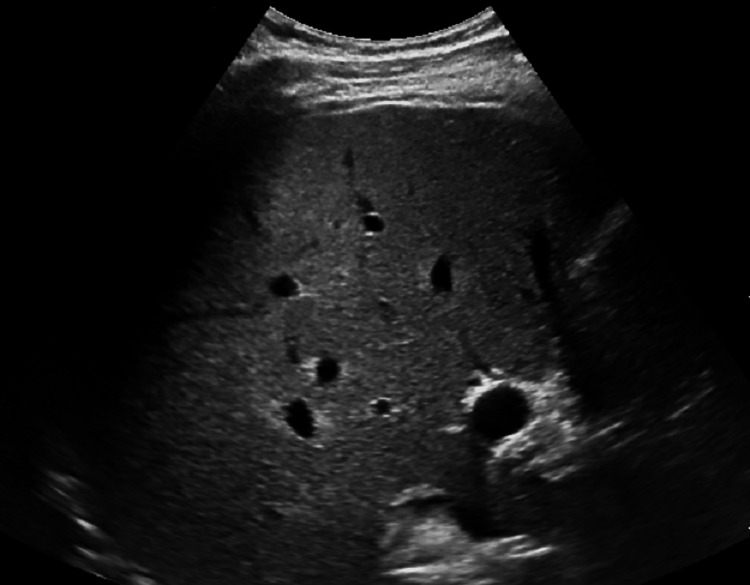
Liver ultrasound showed hepatic steatosis but no cirrhosis.
